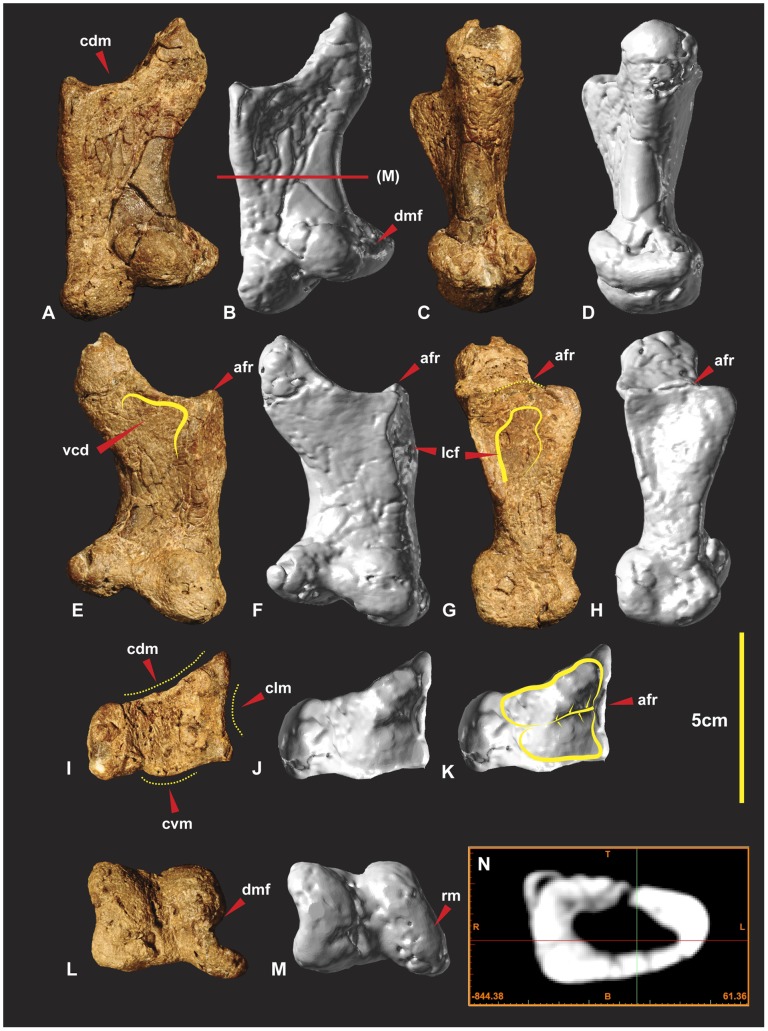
Right Metacarpal I. Right Metacarpal I in dorsal (A & B), medial (C & D), ventral (E & F), lateral (G & H), proximal (I & J), distal (K & L), mid shaft CT image (M). *Abbreviations:* afr, articular facet ridge; cdm, concave dorsal margin; clm, concave lateral margin; cvm, convex ventral margin; dmf, distal medial fossa; lcf, lateral concave facet; rm, repaired margin; vcd, ventral concave depression.
